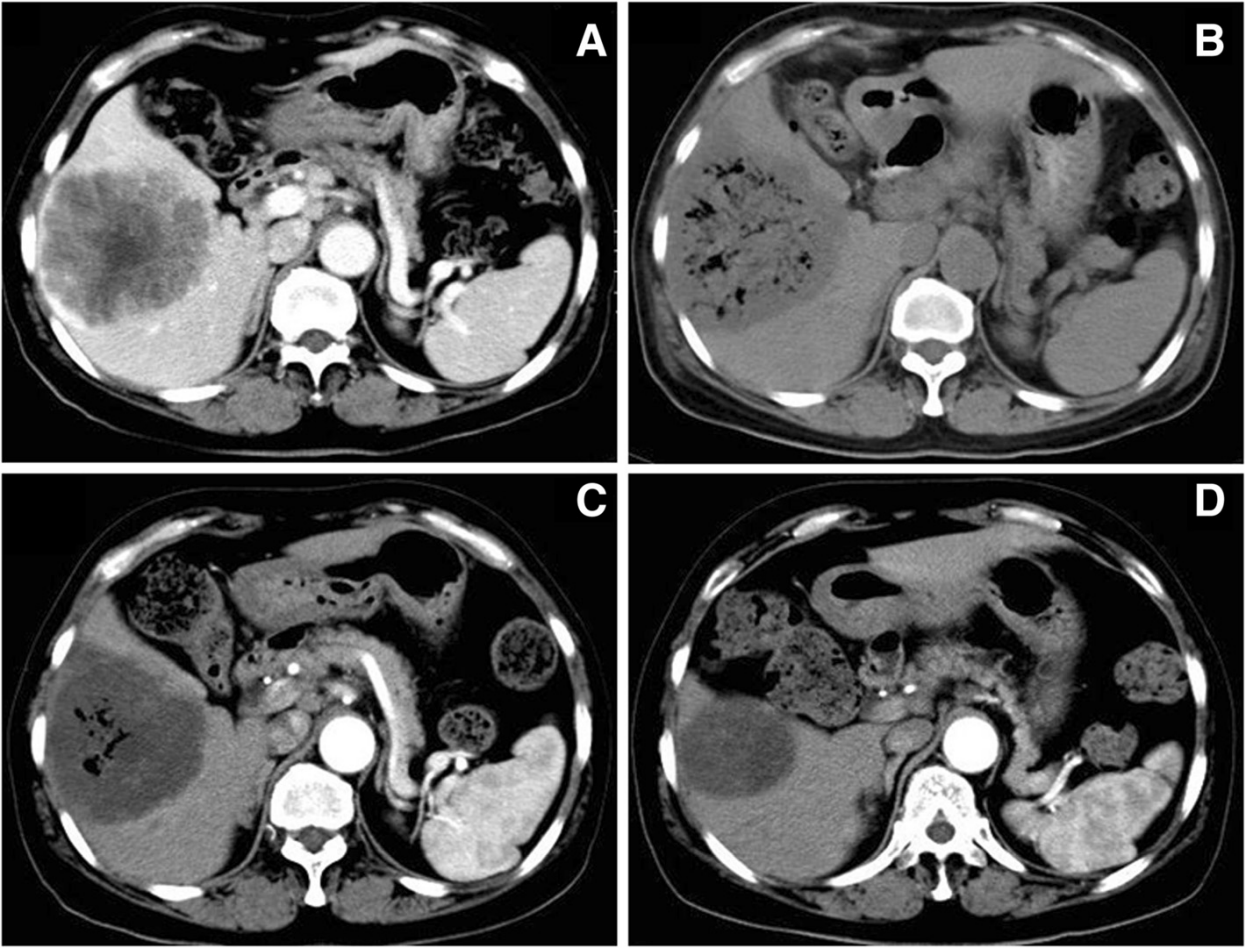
Alteration of tumor lesions before and after chemoembolization by hepatic CT. All images are from the same patient. (A) Tumors showed ring enhancement before embolization; (B) 3 days after embolization, re-examination by CT showed honeycomb-like necrosis of tumor tissue; (C) tumors displayed shrinkage 1 month after embolization, without intensification in the lesions; (D) 3 months after embolization, more profound tumor shrinkage was visible.
